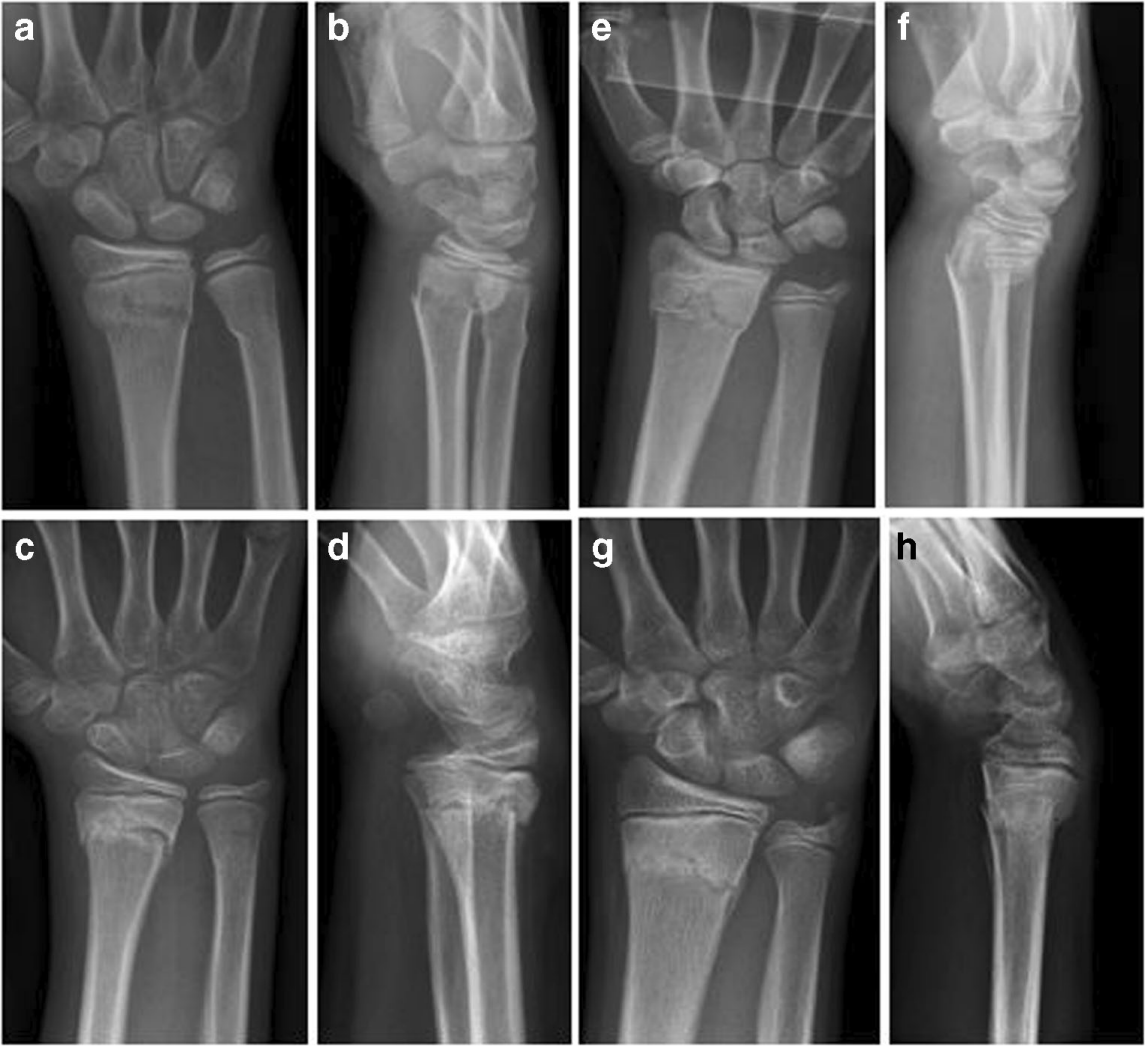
Two cases of metaphyseal fractures with posteroanterior and lateral radiographs at initial presentation before closed reduction and after 28 days. a–d show the X-rays of a 10-year-old female patient treated with a SAC. The initial X-rays before treatment (a, b) and the X-rays after 4 weeks (c, d) are shown. Unrestricted movement of the elbow was possible at the time of cast removal with a normal ROM of the elbow 3 weeks later (extension/flexion 0/0/150°). e–g A 12-year-old male patient treated with a LAC. e and f show the fracture before closed reduction, g and h after 4 weeks of LAC treatment. Restricted range of movement of the elbow for 9 days after cast removal. Three weeks later, ROM of the elbow was 0/0/140°
